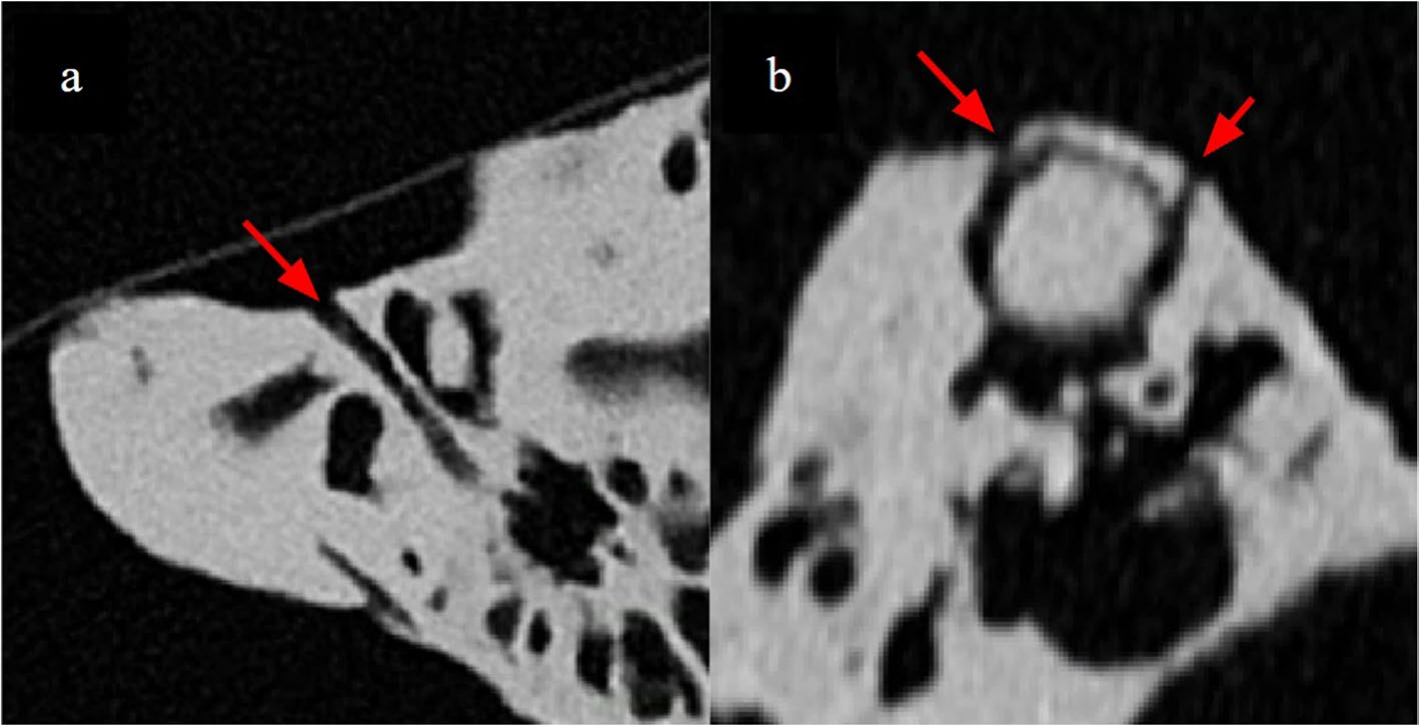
a Non-anatomical resin drainage channels (arrows) created by CAD modelling extending medially from the tympanic segment of the facial nerve, and (b) at the superior aspect of the superior semicircular canal, seen on multiplanar reconstructed CT imaging. These channels are placed in locations to allow millifluidic post processing (see step: 3D printing and post processing) while not interfering with surgically important anatomical landmarks
